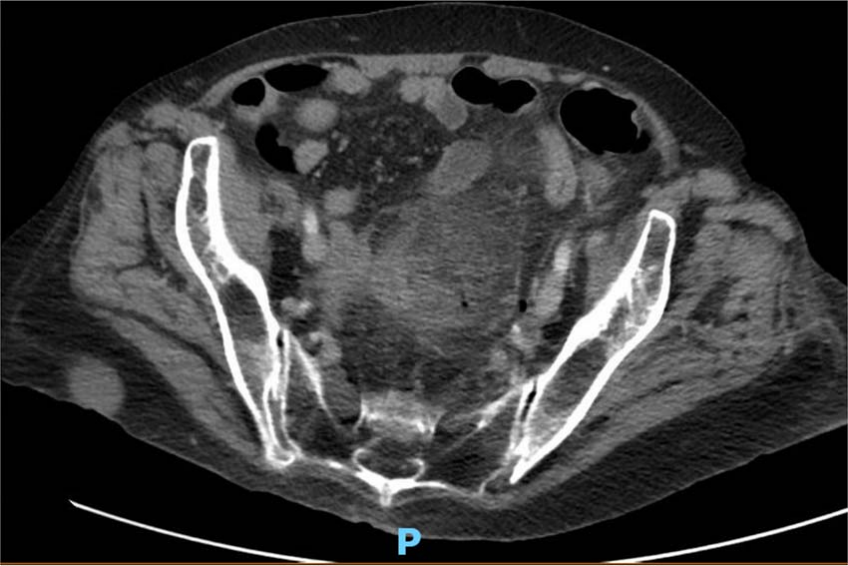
CT with perforation from prior admission.
